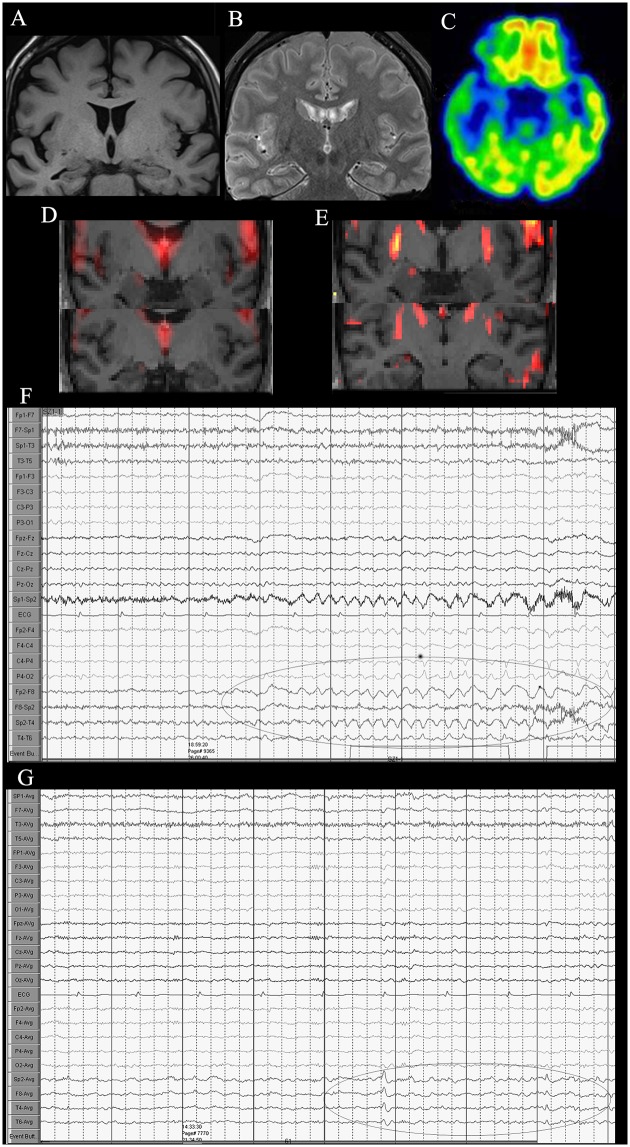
A 42-year-old woman diagnosed with right mesial temporal lobe epilepsy. (A) Coronal T1-weighted image showing right hippocampus atrophy, (B) FLAIR image showing slightly high signal of right hippocampus. (C) ^18^F-FDG PET/CT imaging reveals low FDG uptake in the left temporal lobe region, particularly, in the hippocampus. (D) Coronal ALFF and (E) ReHo reveal abnormal activation in the right hippocampus compared to the left side. (F, G) VEEG showing ictal and interictal discharges in the right temporal lobe.
